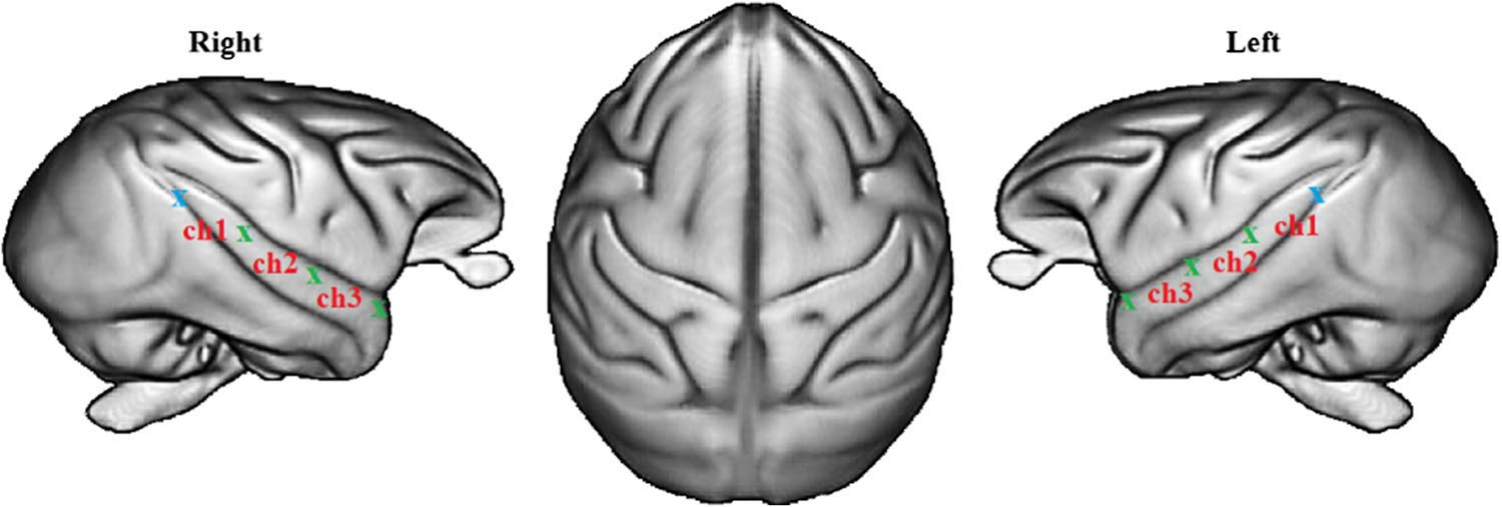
fNIRS optode and channel locations according to 89 baboons T1 MRI template (Love et al., 2016). Blue and green crosses represent optical receivers and transmitters, respectively. Ch1, Ch2, and Ch3 indicate the three channels on the right and left temporal cortex
